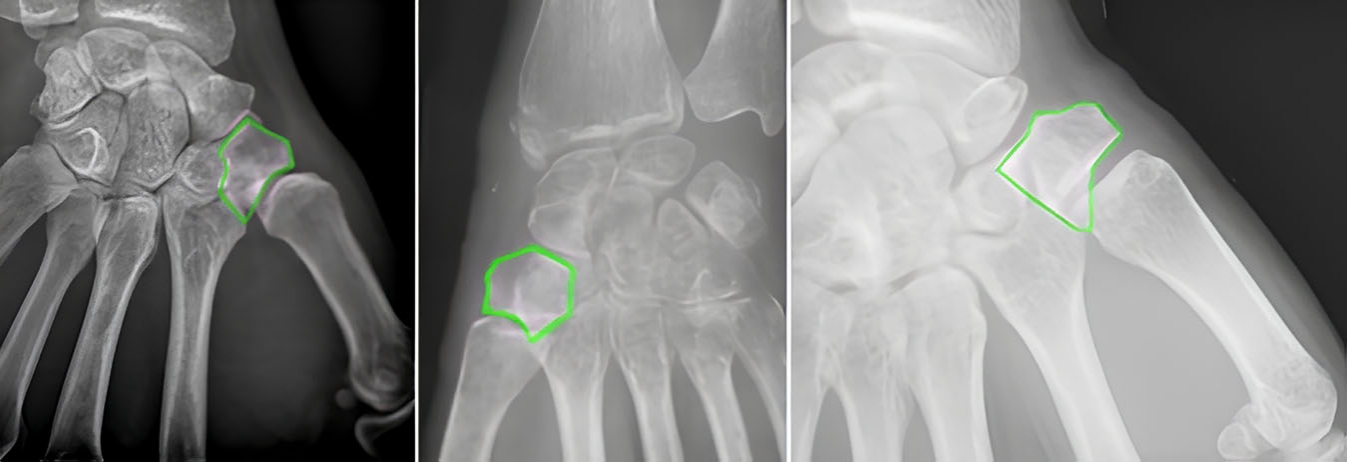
Radiograph from dataset (Hôpital Avicenne, Assistance Publique-Hôpitaux de Paris, Bobigny, France, anonymized image) showing the trapezium (green box). The bone is partially obscured by surrounding structures, highlighting the need for automated localization. Inter-observer agreement was excellent, with Cohen κ = 0.89 and DSC = 93.8%
